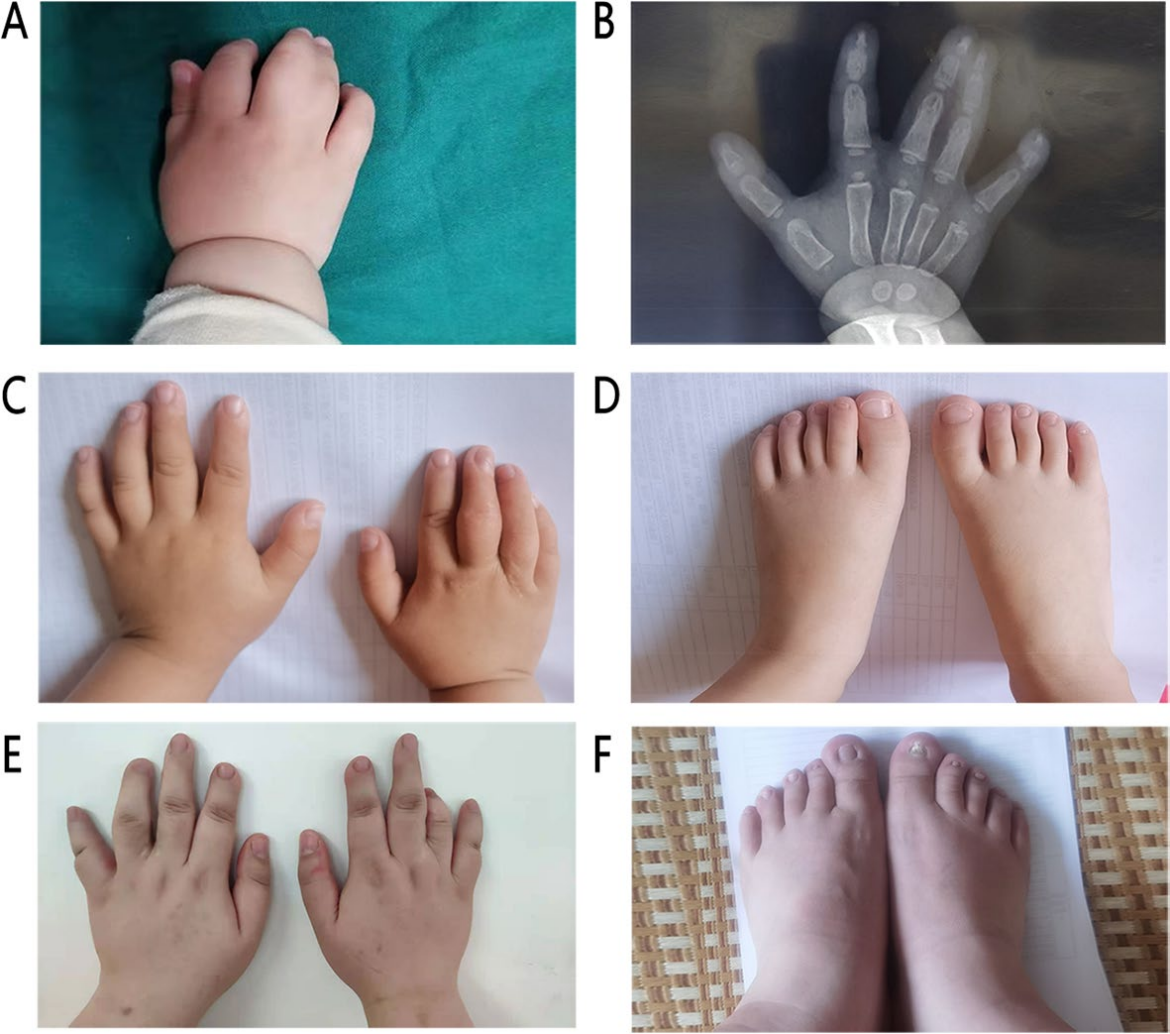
Phenotype of the proband and her mother. a A photograph of the proband's right hand before surgery. b An X-ray of the proband’s right hand before surgery. c A picture of the proband’s hands after the operation. d A photograph of the proband's feet. e A photograph of the mother’s hands after the operation. f A photograph of the mother’s feet
